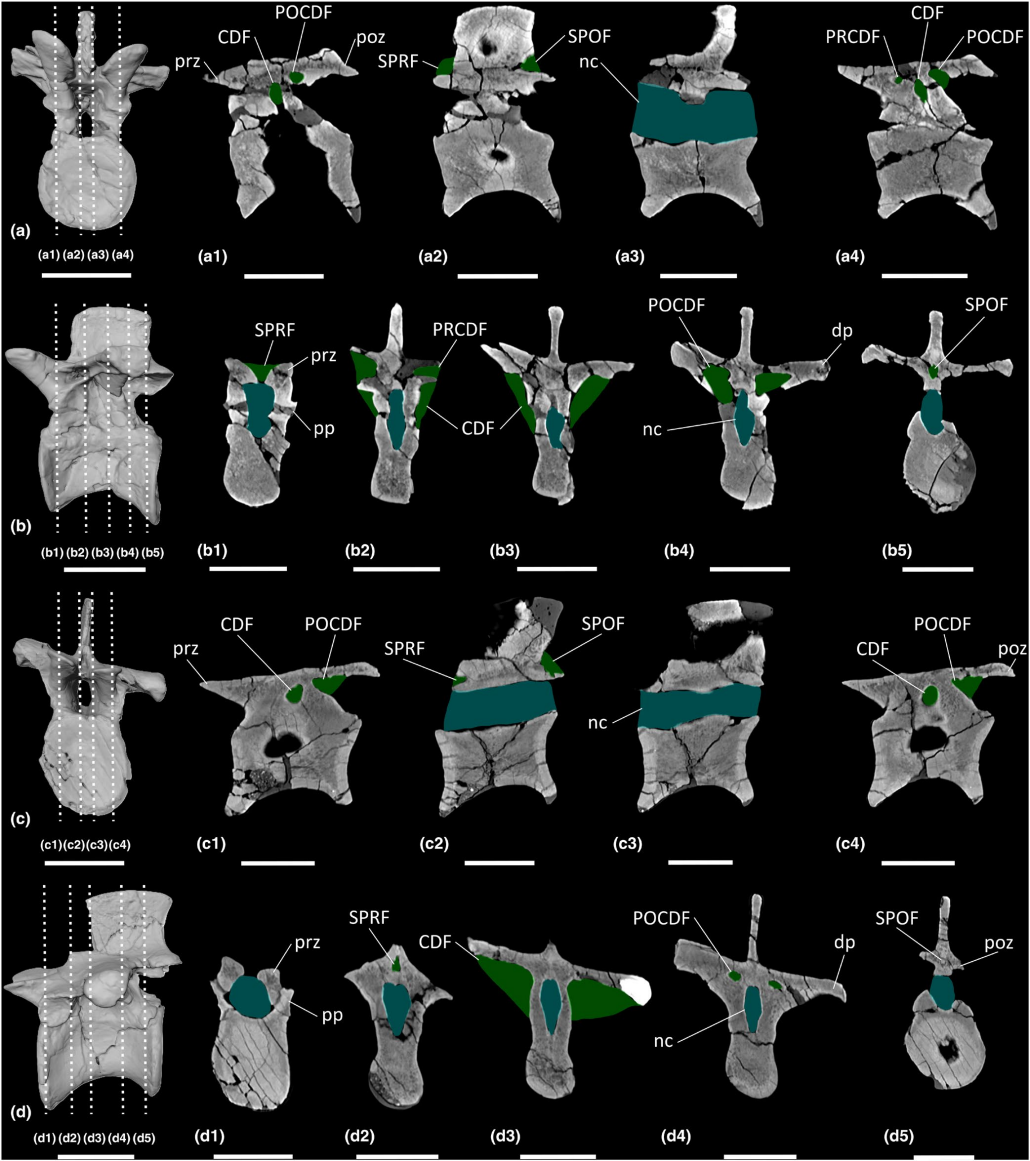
CT scan data of *Plateosaurus longiceps* MB.R.4404 ‘Skeleton 25’ middle dorsal vertebrae. (a, b) dorsal vertebra 6 MB.R.4404.30, (c, d) dorsal vertebra 10 MB.R.4404.34; (a, c) surface scan anterior view, (b, d) surface scan lateral view; (a1–a4, c1–c4) CT scan slices in parasagittal cross‐section, (b1–b5, d1–d5) CT scan slices in transverse cross‐section. CDF, centrodiapophyseal fossa; dp, diapophysis; nc, neural canal; POCDF, postzygapophyseal centrodiapophyseal fossa; poz, postzygapophysis; pp, parapophysis; PRCDF, prezygapophyseal centrodiapophyseal fossa; prz, prezygapophysis; SPOF, spinopostzygapophyseal fossa; SPRF, spinoprezygapophyseal fossa. Key: Blue highlight, neural canal; green highlight, fossa. Scale bars = 5 cm.
